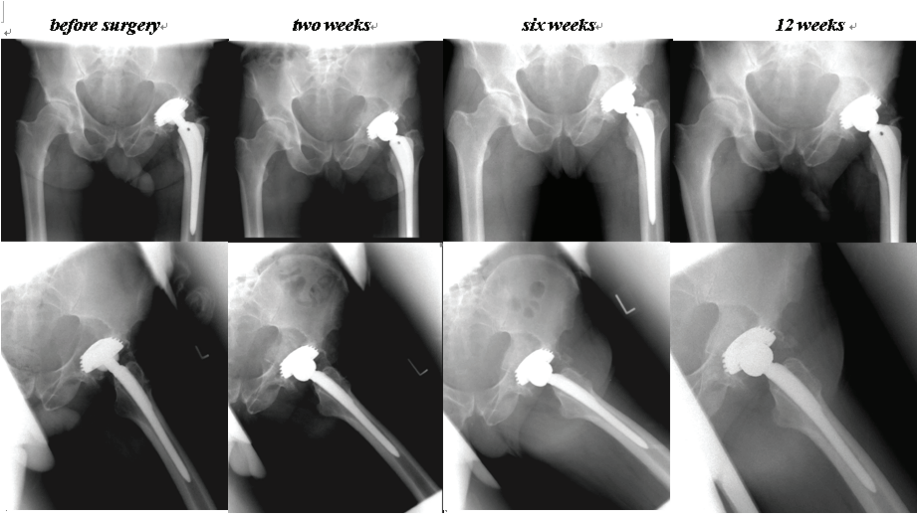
The figure shows x-rays in two standard planes of pelvis/hip. The roentgenological follow-ups before surgery and after two, six and 12 weeks after BMP-2/MSC-tretament show a progressive bony healing of the osteolytic area behind the cup.
